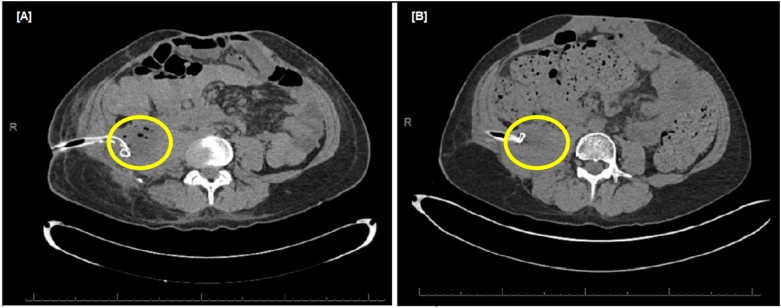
[A] CT scan shows percutaneous drain in right retroperitoneal infected collection (yellow ellipse) prior to VARD. [B] Resolution of the abscess cavity (yellow ellipse) with drain in place following VARD procedures. (For interpretation of the references to colour in this figure legend, the reader is referred to the web version of this article.)
